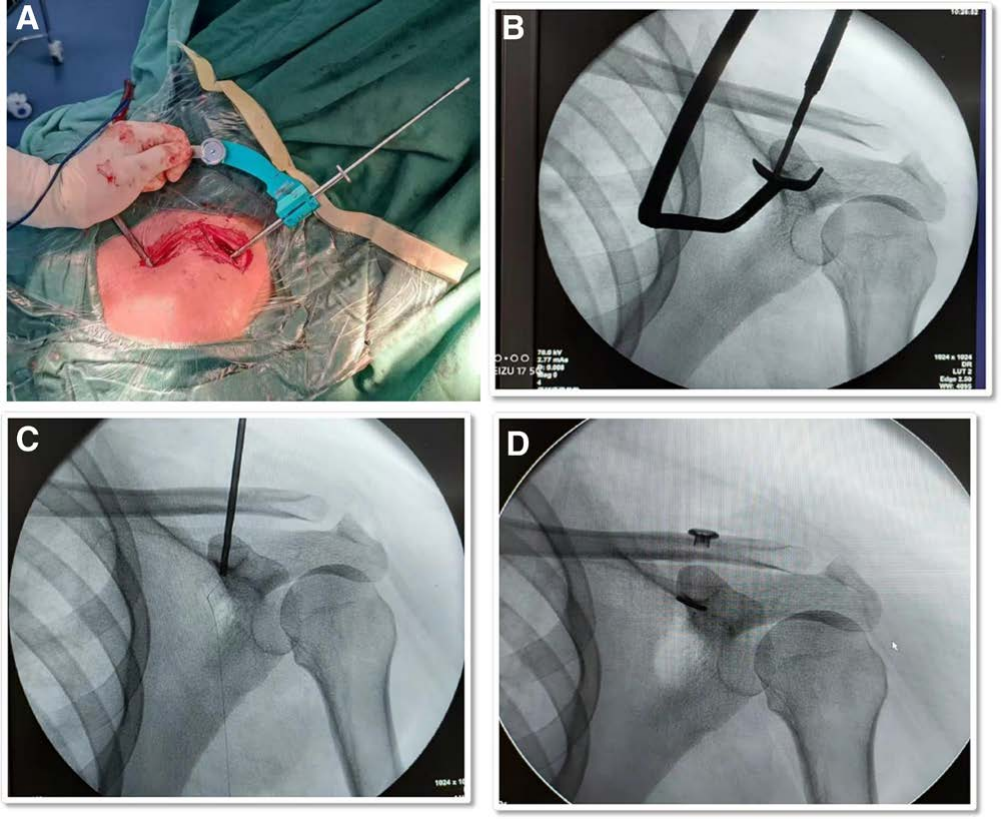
The picture and X-ray of a 49-year-old man with Rockwood type IIIB ACJ injury treated by the low-profile loop titanium plate system. Panel (A) shows the surgical incision and localization of the coracoid positioner; (B) shows X-ray image of Coracoid positioner aligned with the base of the coracoid process under fluoroscopic guidance; (C) shows X-ray image of the establishment of bone tunnels; (D) shows X-ray image of anatomical reduction with the low-profile loop titanium plate system. ACJ = acromioclavicular joint.
